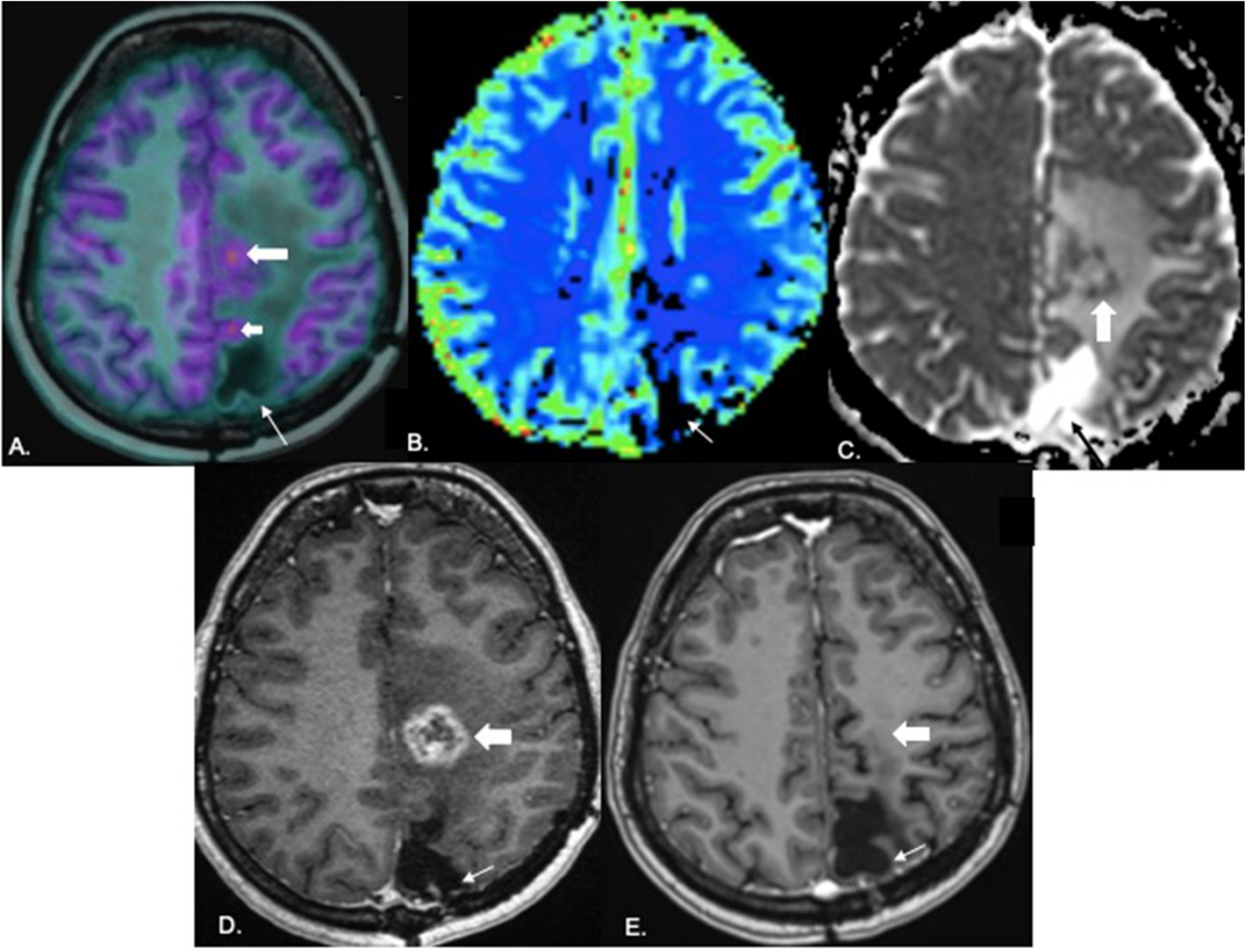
False positive diagnosis on PET: 34-year-old female diagnosed with anaplastic ODG 2 years back with enhancing lesion on MRI. PET image fused with axial T1 MPRAGE (A) shows an area of uptake along the parasagittal region of the left frontal lobe (large thick arrow) and another area (small thick arrow) along the margin of the resection cavity (linear white arrow) raising a suspicion of recurrence. There is no evidence of elevated perfusion on the rCBV map (B). Resection cavity is shown by white arrow. Peripheral diffusion restriction is seen along the margins of the lesion in the left centrum semiovale (block white arrow in C). There is no restriction along the resection cavity (black arrow). Peripheral enhancement is seen along the margins of the lesion in the left centrum semiovale (block white arrow in D) with no enhancement along the resection cavity (white arrow). One month follow-up axial post-contrast T1 MPRAGE (E) shows the resection cavity (thin white arrow) with complete resolution of enhancement in the left centrum semiovale (block white arrow) suggestive of treatment related changes excluding recurrence
